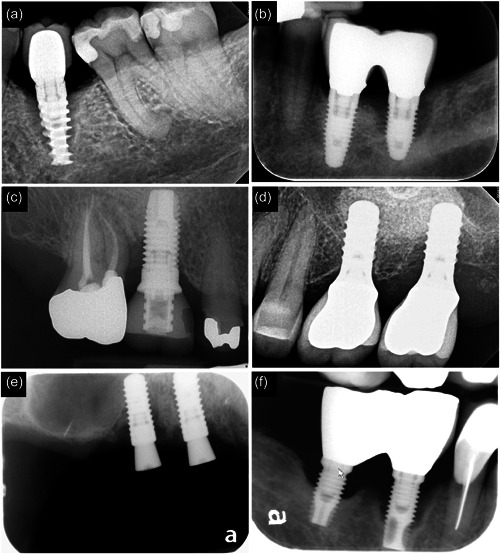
Radiographic images of a set of implants removed due to severe bone loss. These implants represent different thread type (aggressive [a], regular [b, c, e, f] or mild [d]), surface characteristics (oxidized [a–c, f] or SLA [d, e]), level of placement (bone [a–c, e, f] or tissue level [d]) and abutment fit (butt joint [b–d, f] or platform‐shifting [a, e]) and splinted (b and f) and nonsplinted (d and e) adjacent implants. SLA, Sand‐blasted, large grit, acid‐ etched.
